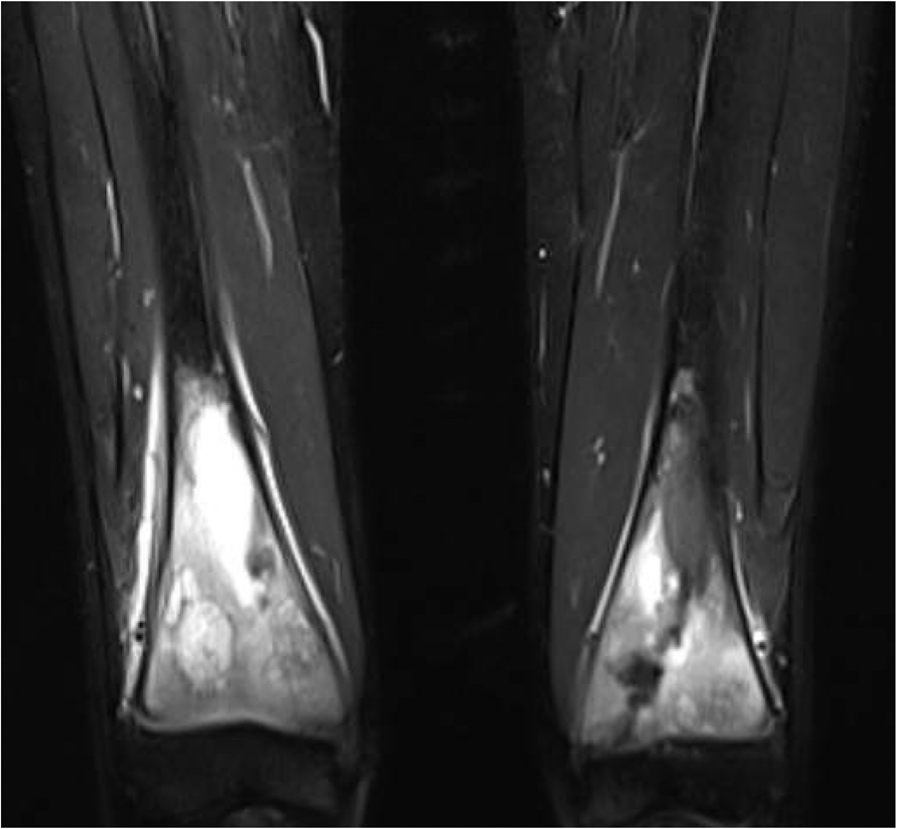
Lower Extremity MRI (STIR) showing multifocal distal femoral abnormalities with regional bone marrow edema pattern, cortical thickening, and periostitis
